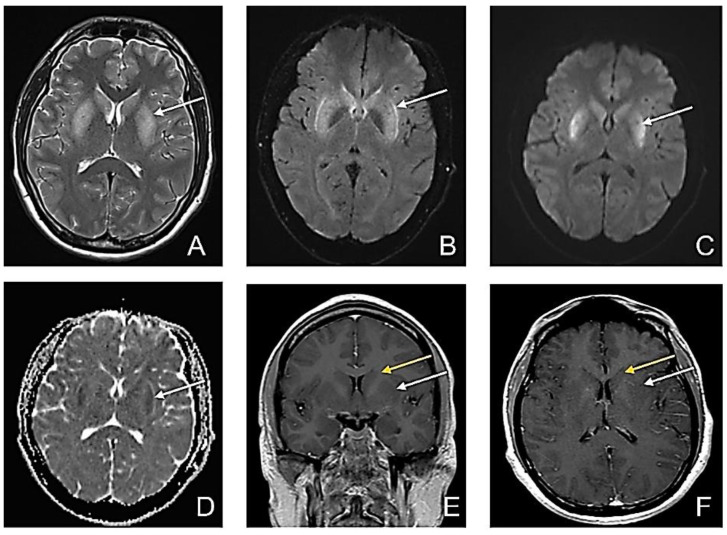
Second MRI-D5. (A) Axial T2-weighted image sequence: bilateral putaminal high signal intensity; (B) Axial FLAIR sequence: bilateral putaminal high signal intensity; (C) Axial DWI: high signal intensity in putaminal regions; (D) Axial ADC map: low ADC in the putaminal regions suggestive of restricted diffusion in both putaminal regions (white arrow); (E) Contrast-enhanced Coronal T1wi; and (F) Contrast-enhanced Axial T1wi: no enhancement in the bilateral caudate nucleus (yellow arrow) and putamen (white arrow) with slightly decreased signal in bilateral putamen.
